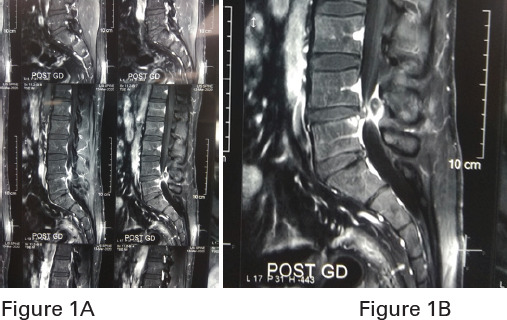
MRI of LS spine showing unusual disc protrusion at L4-L5- 1A: plane, 1B: contrast.
